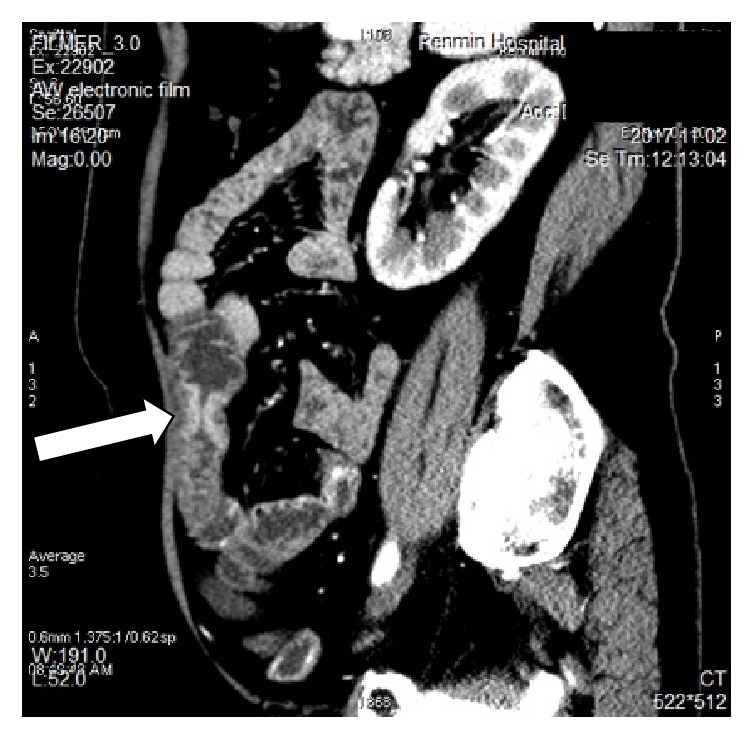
CT scan showing thickened intestinal wall and calcification.
